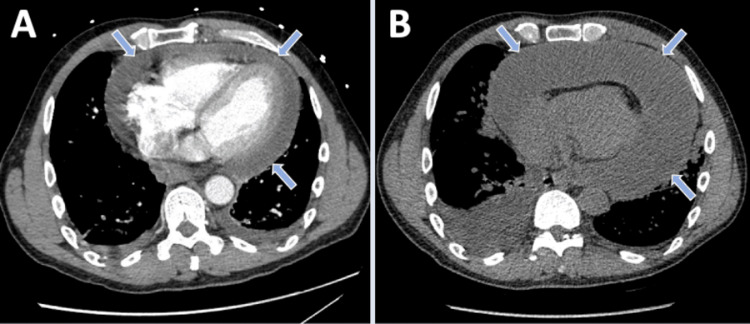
Chest computed tomography findings (A) Chest CT from index admission showing moderate-sized pericardial effusion (arrows). (B) Chest CT from the second admission with large pericardial effusion increased in size from the previous (arrows).
